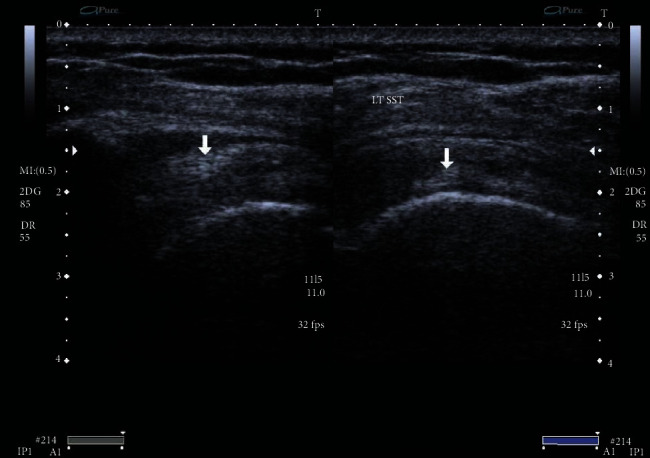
Ultrasonographic changes in calcified supraspinatus tendon (RPT group).
